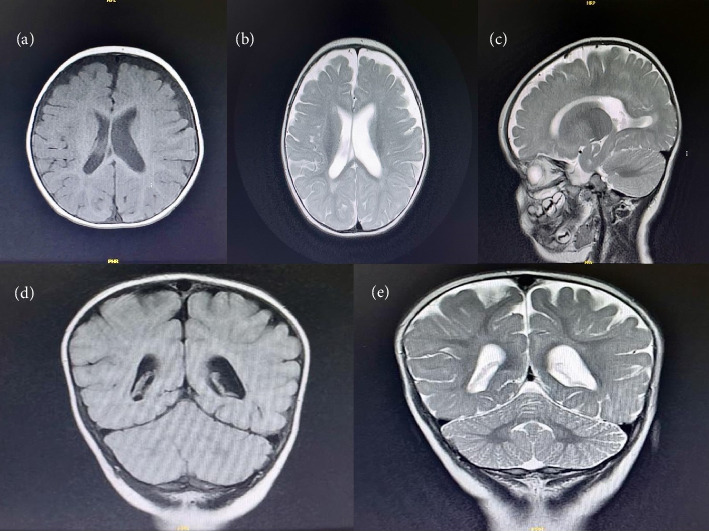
Brain MRI findings: (a and b) Axial T1- and T2-weighted images demonstrate reduced white matter volume along the bilateral lateral ventricles, with more pronounced changes at the trigones. (c) Sagittal T2-weighted image reveals prominent cortical sulci and enlarged extra-axial cerebrospinal fluid spaces, suggestive of cerebral atrophy. (d and e) Coronal T2 FLAIR and Coronal T2 images show hyperintense signal changes, indicating underlying white matter abnormalities.
